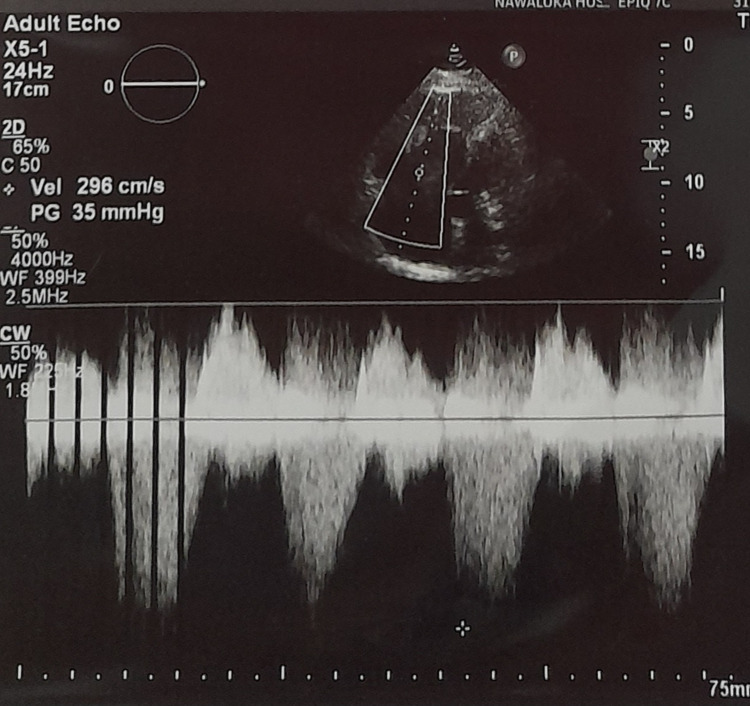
Transthoracic echocardiogram demonstrating a grossly dilated right atrium and right ventricle
